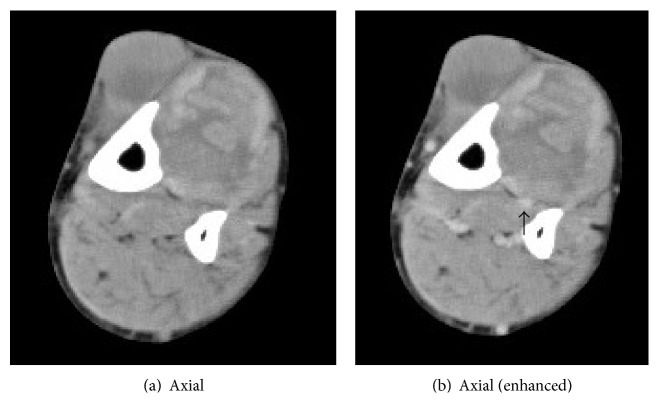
Computed tomography (CT) of left lower leg revealed a large mass (maximum diameter, 7.2 cm; length, 21.0 cm) with cystic lesions in the region of the TA and EHL muscles (a and b). The mass and cystic lesions were not contrasted but the anterior tibialis artery was clearly observed (arrow) by using enhanced CT (b).
